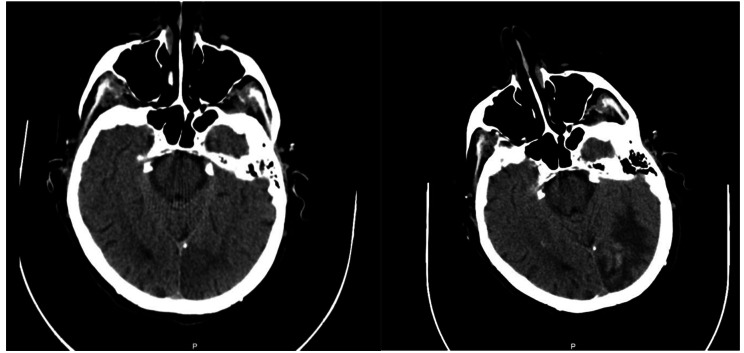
81 year old female with a history of peripheral vascular disease presenting with toe pain in bilateral feet. (A) Axial CT head 26 days prior to ED visit demonstrating loss of gray-white differentiation in the medial left occipital lobe, consistent with acute to subacute left PCA territory infarction. (B) On presentation Axial CT head demonstrates evolution of a left occipital subacute infarction, larger and more discrete appearing than previously, also now with petechial hemorrhage
